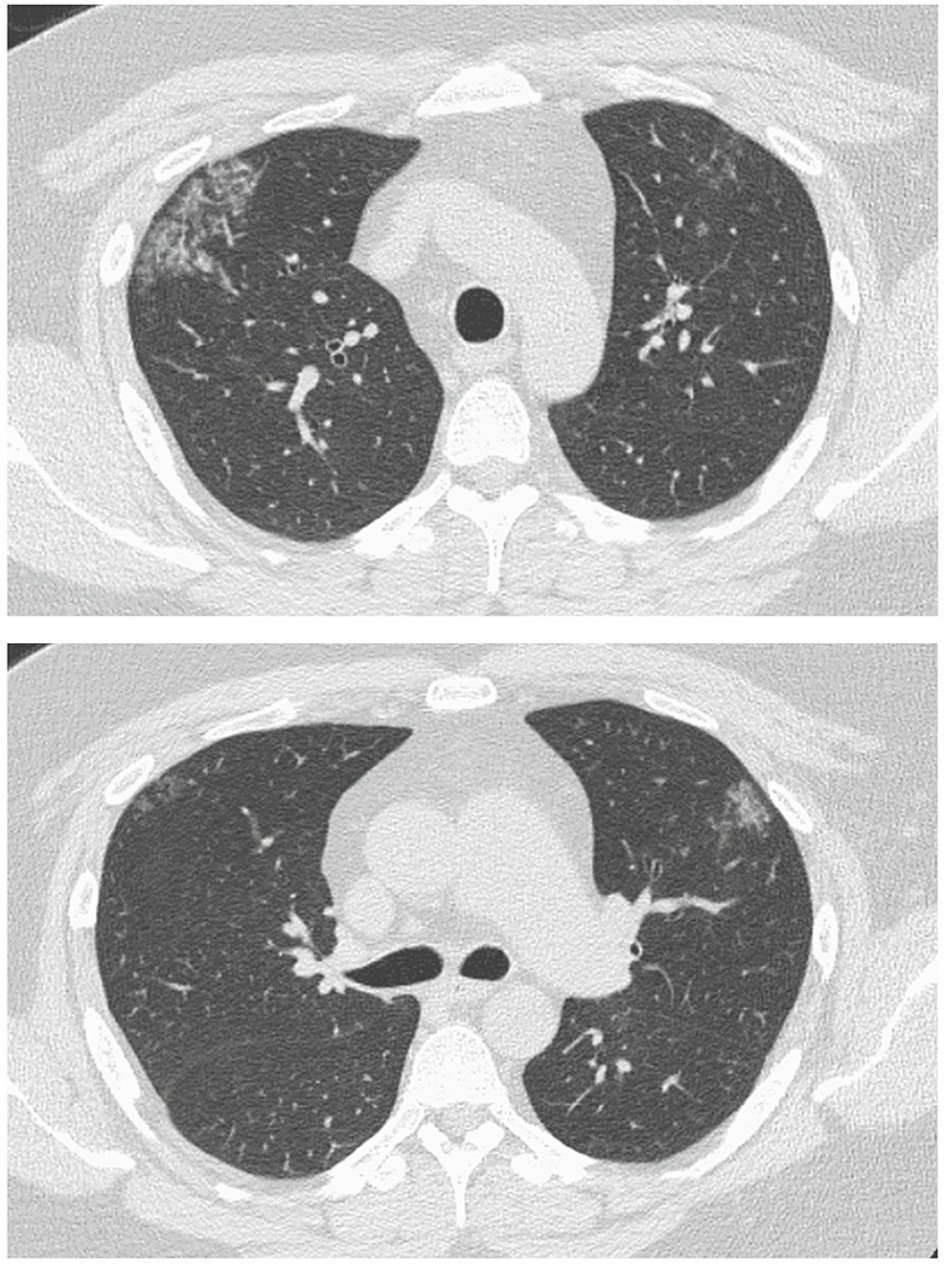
CT in January 2022 showed ground-glass opacities and infiltrative shadows in the subpleural area of both upper lobes, which were exacerbated in comparison to January 2020. CT, computed tomography
